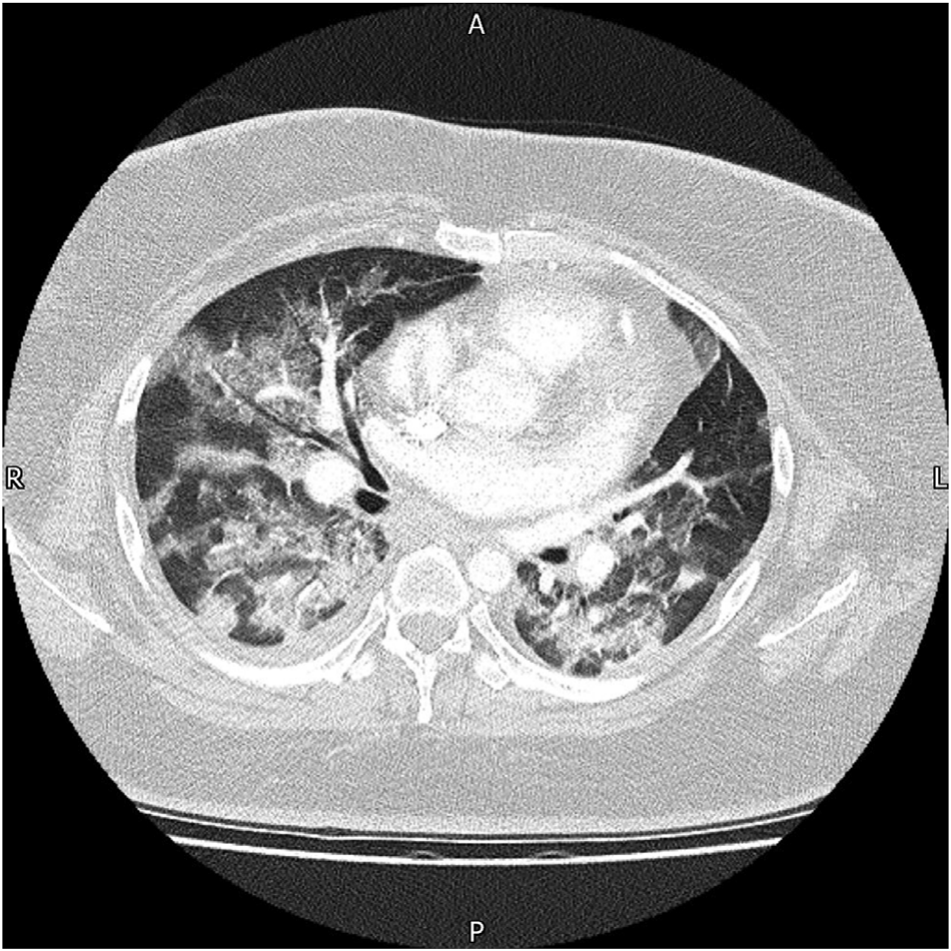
CT imaging of a 34-year-old female, who required intubation and ventilation for severe type 1 respiratory failure due to COVID-19. Bilateral ground glass opacification is demonstrated.
